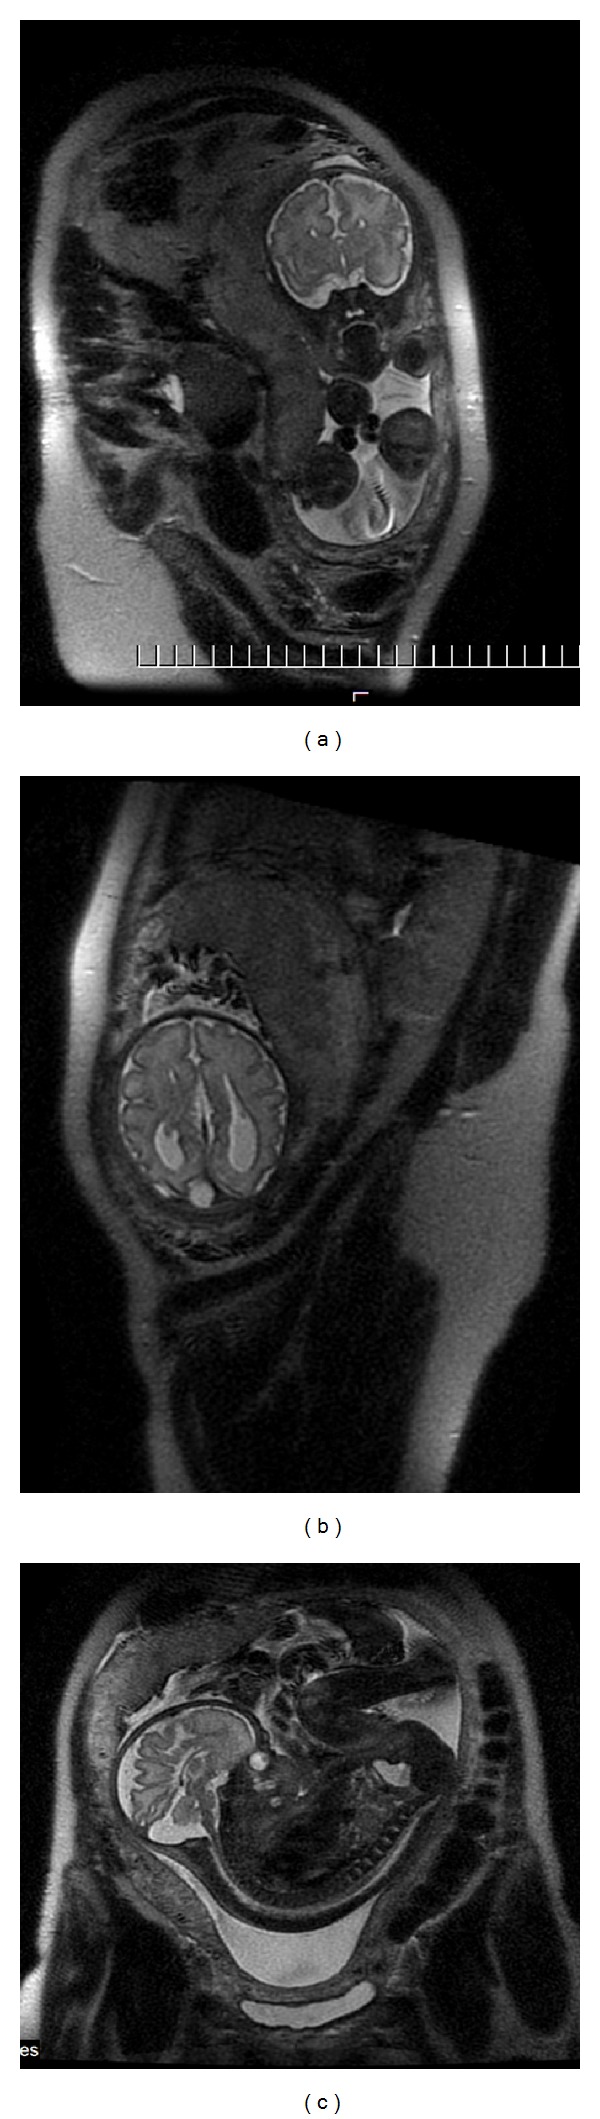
MRI of fetal brain. SSFSE sequence, T2-weighted images (T2WI). (a) Wide hemispheric fissure, and “viking helmet” appearance of the lateral ventricles on a coronal image. (b) “Tear drop” configuration (colpocephaly) as a result of enlargement of occipital horns of the lateral ventricles in the axial projection. (c) “Sunray” appearance of brain sulci in the midsagittal plane.
